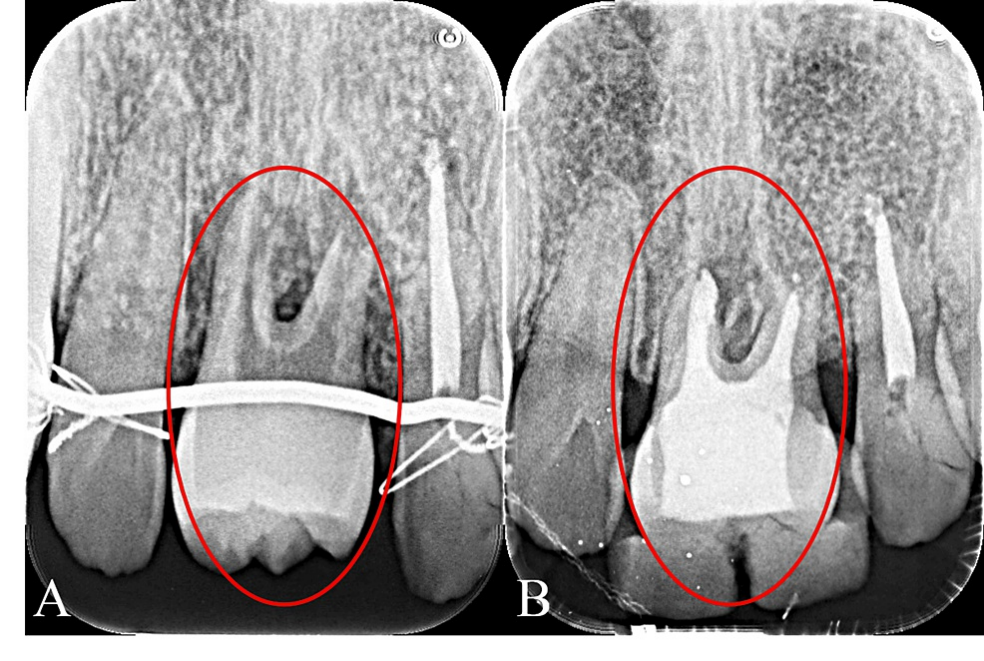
Dental X-ray after autotransplantation (A) Immediately after autotransplantation. (B) After root canal treatment. The circle shows the autotransplanted tooth.
